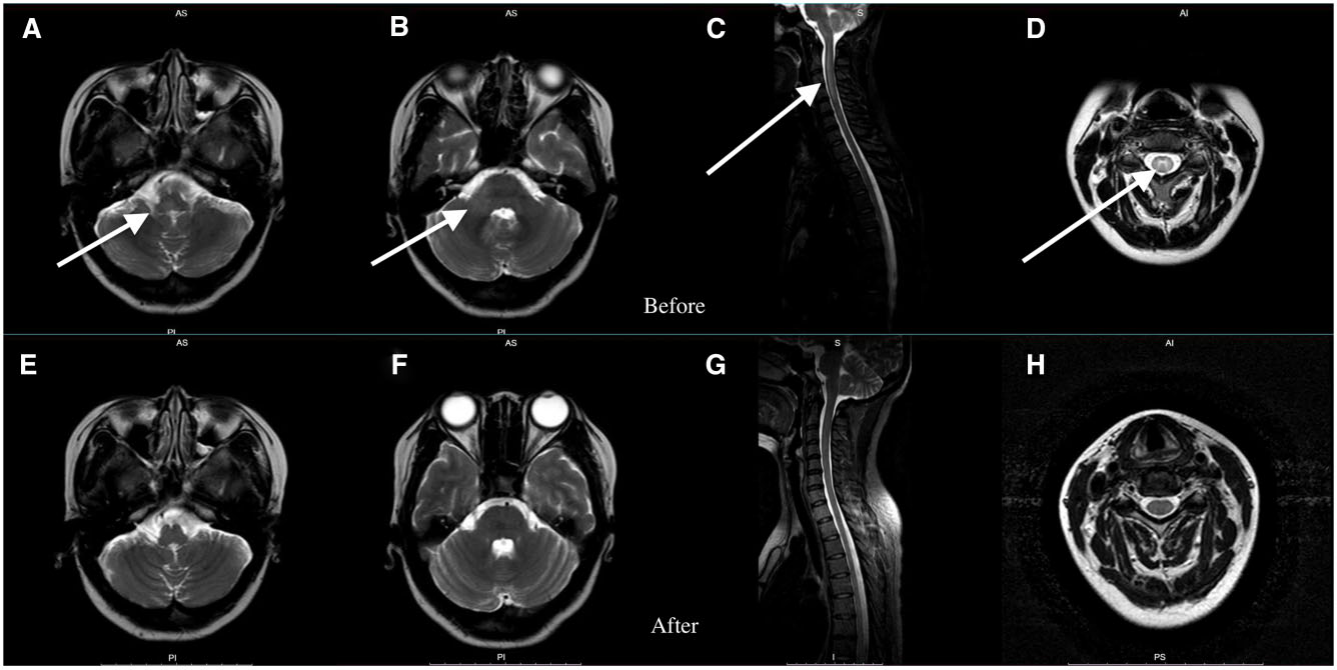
Brain DWIs and full-spine MRI using T2-weighted signal enhancement. (A) The medulla oblongata. (B) The middle cerebellar peduncle. (C) Cervical spinal cord thickening. (D) ‘H’-shaped enhancement in the central region of the spinal cord. (E–H) The same images after treatment
